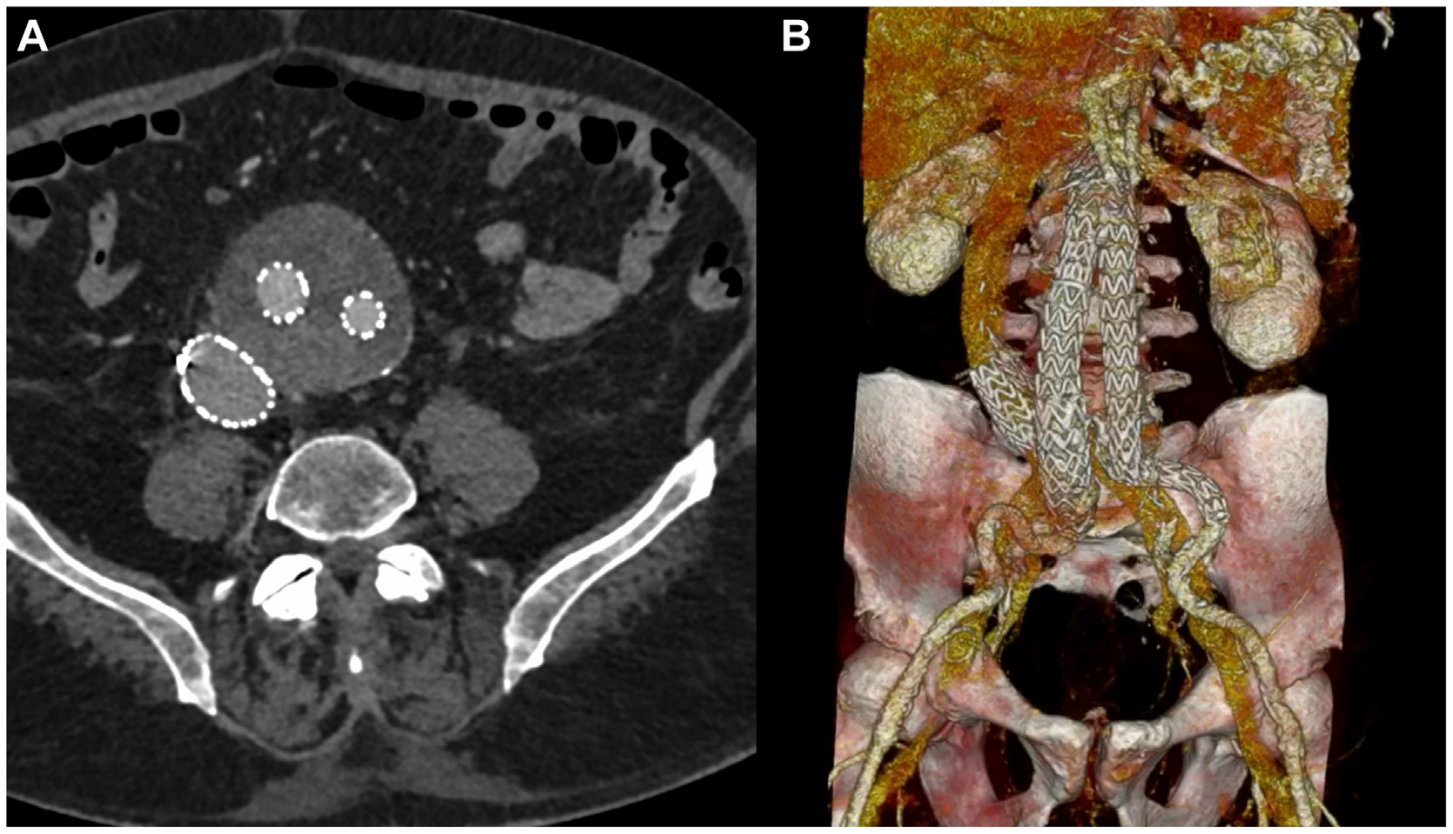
Follow-up computed tomography angiography (CTA) at 24 months postoperatively showing no signs of endoleak or aortocaval fistula (ACF; A) and perfect patency of all endografts implanted (B).
